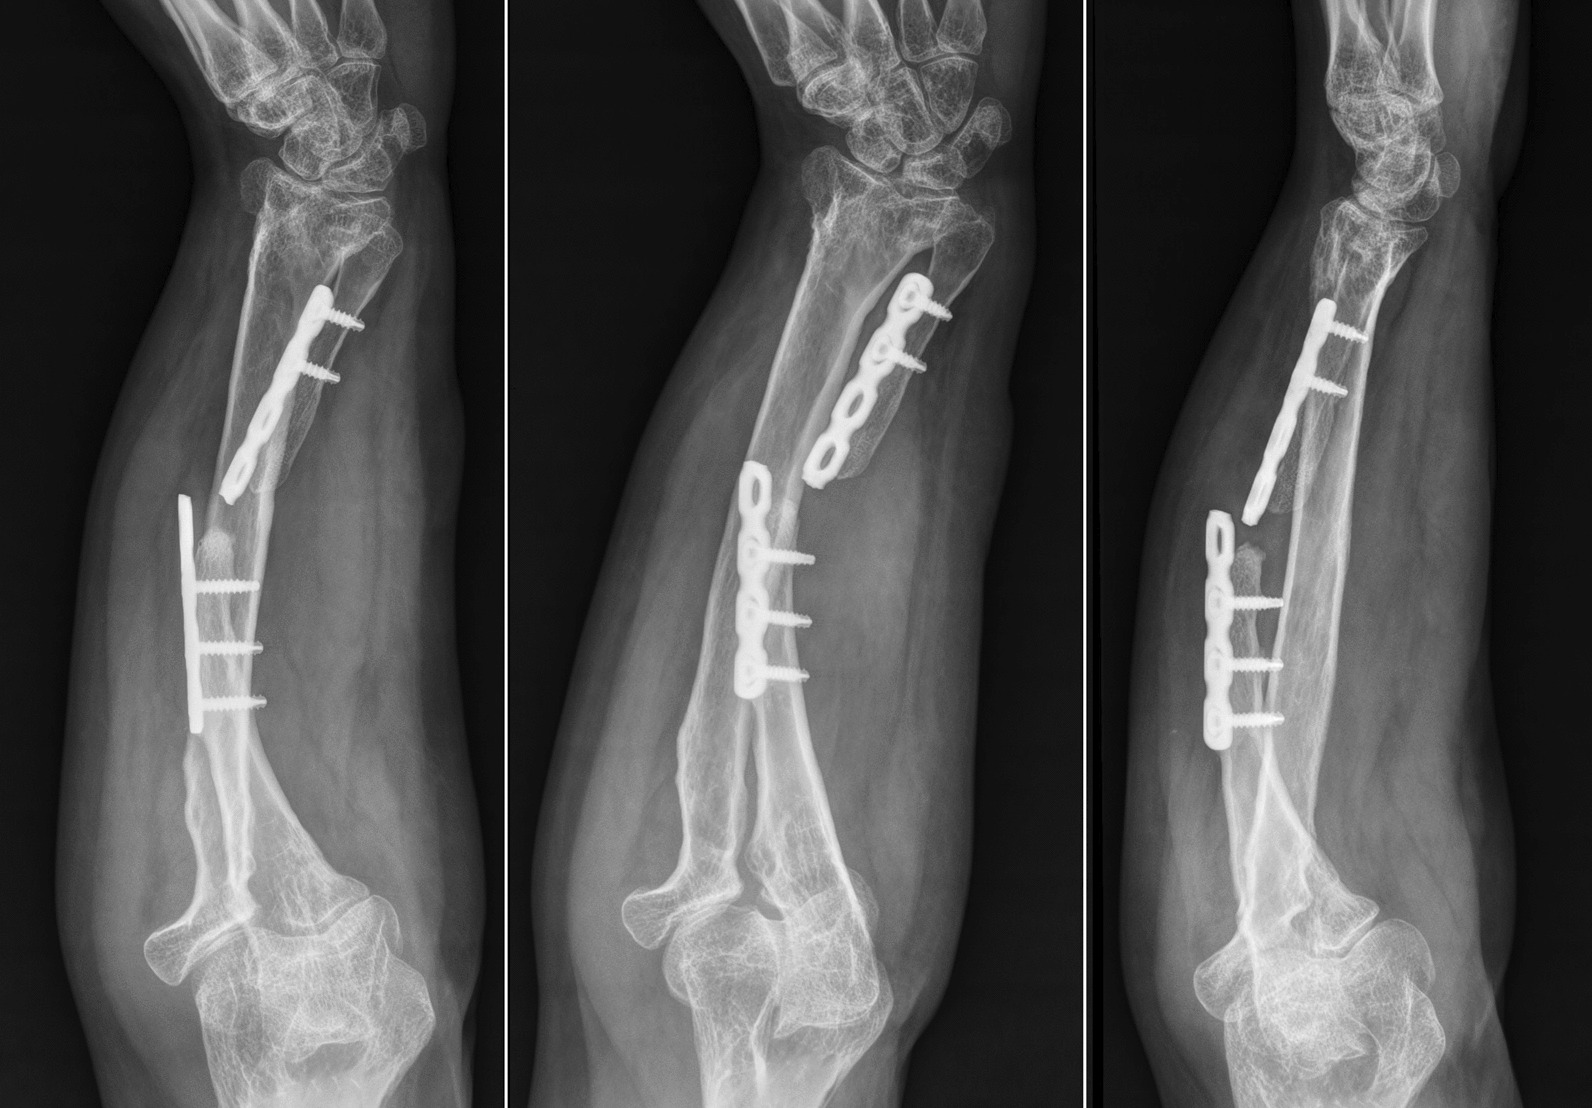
Last pre-op X-rays showing non union shaft site with evident bone graft loss and plate breakage
